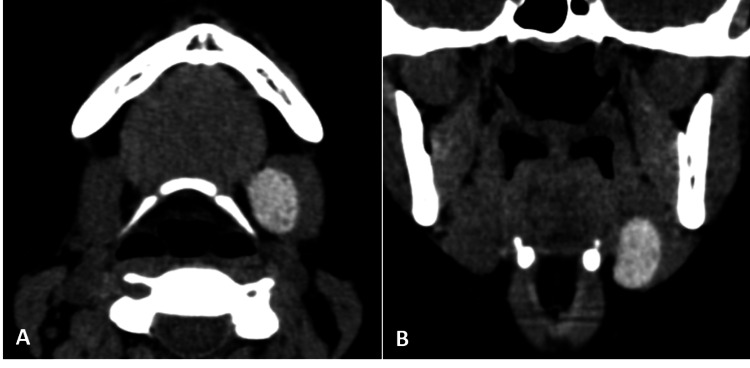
(A, B): CT images showing a well-defined, oval, homogenously hyperdense lesion in the left submandibular region compressing the submandibular gland and displacing it laterally.
